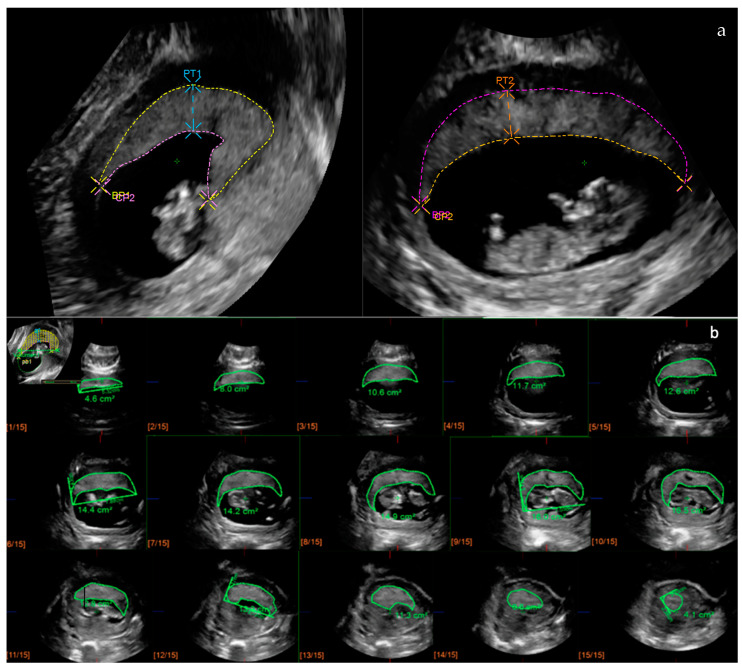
Measurement of placental biometry with 2D ultrasound and 3D ultrasound. (a) All variables were measured at the largest view of the placenta in two orthogonal planes. BP, basal plate; CP, chorionic plate; PT, placental thickness. (b) Volume calculation using QLAB GI3DQ software (15 sections). Each frame depicts the contoured area, manually traced, of the 15 sections of the placenta. PV, placental volume; 2D, three-dimensional; 3D, three-dimensional.
